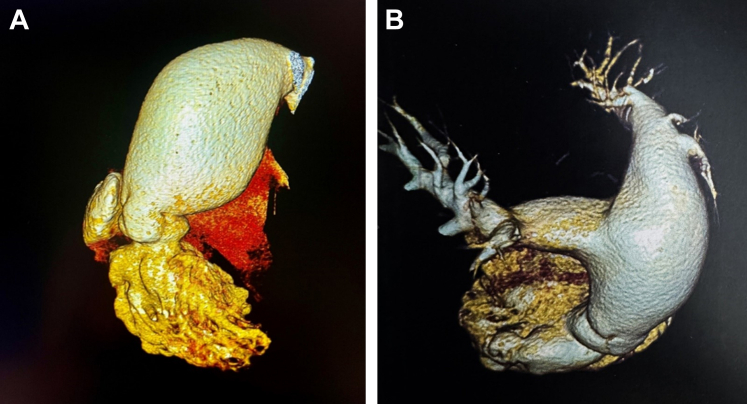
Computed Tomography Angiography of the Pulmonary Artery (A and B) Significant enlargement of the main pulmonary artery is evident. Although the right pulmonary artery and left pulmonary artery appeared normal at the hilum, their diameters at the ostium suggest aneurysmal changes. Clear dilation of the main pulmonary trunk is also observed.
